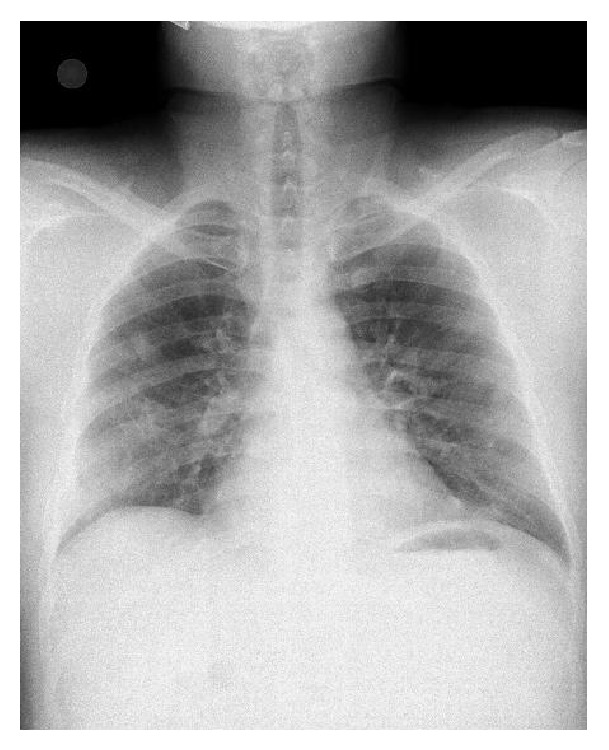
Regression of nodular lesions at the postoperative 2nd month follow-up chest X-ray following chemotherapy.
